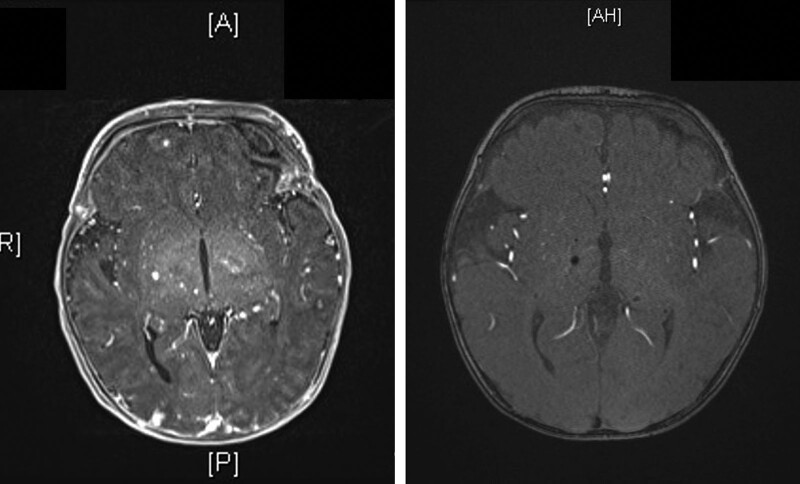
Brain MRI after 2 wk of birth showed bilateral cerebrum and cerebellum cavernomatosis (A) and regressed after 6 mo treatment of sirolimus (B). MRI = magnetic resonance imaging.
